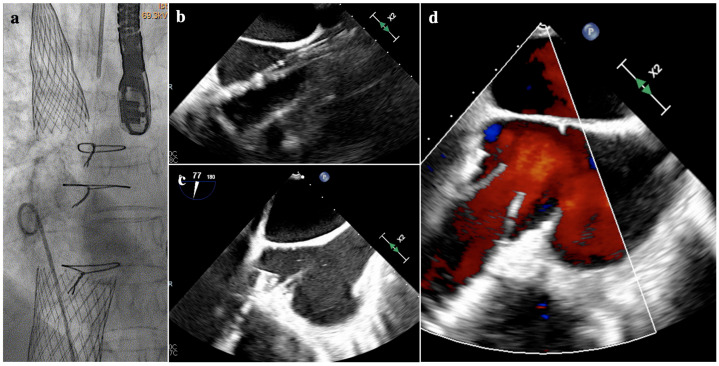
An example of TricValve implantation with a 29 mm prosthesis in the SVC and 35 mm prosthesis in the IVC (a). TEE monitoring can be challenging for the superior prosthesis, and visualization is often suboptimal (b). The TEE can easily visualize inferior prosthesis delivery (c) and evaluate valve function and positioning (d). IVC: inferior vena cava; SVC: superior vena cava; TEE: transesophageal echocardiography.
